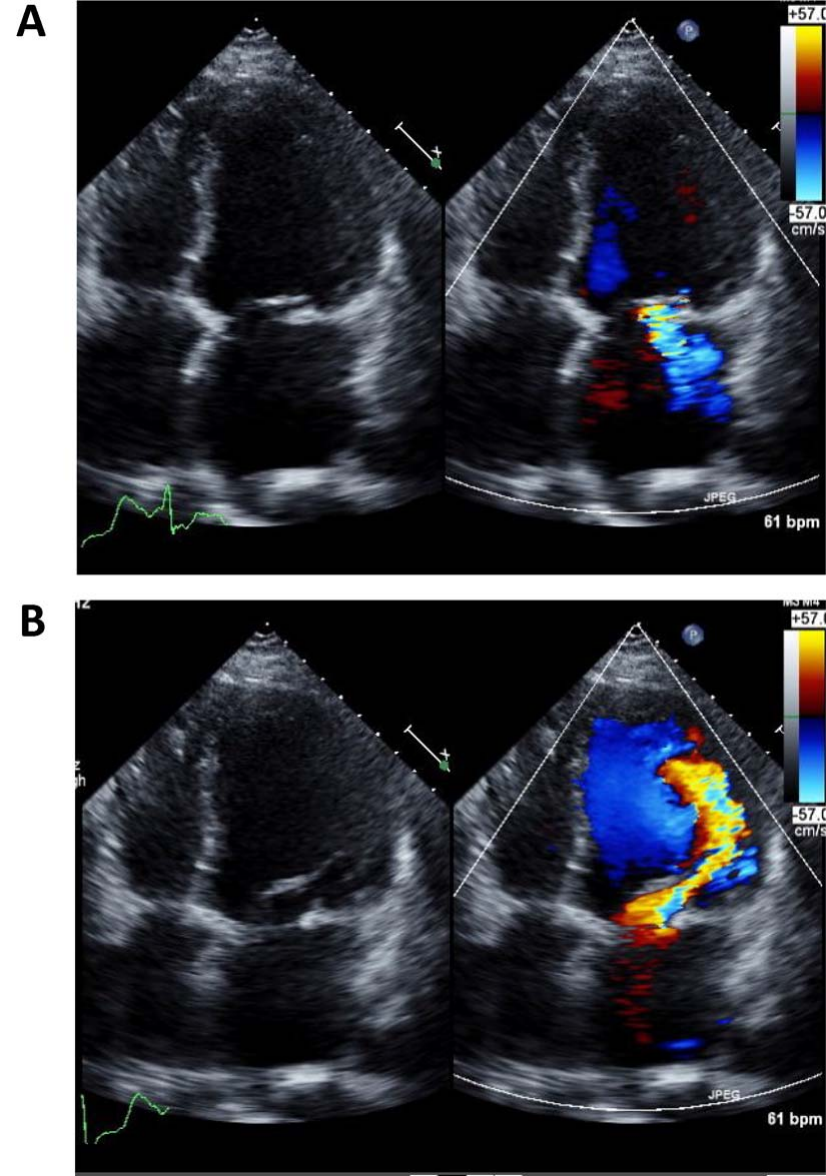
Preoperative echocardiography of left ventricle in systolic (A) and diastolic (B) phases.
